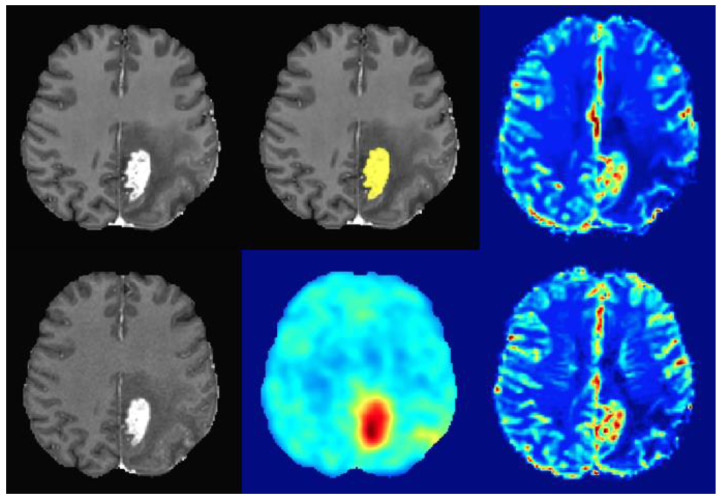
Exemplary images of a 57-year-old female patient with left parietal glioblastoma (upper left: MRI contrast-enhanced T1, upper middle: MRI contrast-enhanced T1 with automated segmentation overlay (yellow: CET), upper right: MRI CBV, lower left: PET/MRI contrast-enhanced T1, lower middle: FET-PET, lower right: PET/MRI CBV).
